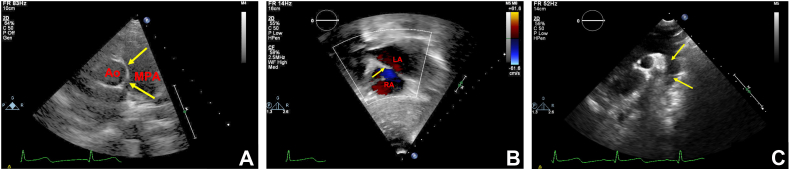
(A) Two-dimensional TTE (5 MHz transducer) high right parasternal view demonstrates intact septation between the aorta and MPA *(arrows)*. (B) Subcostal coronal view with color flow Doppler demonstrates the intact atrial septum *(arrow)*. (C) Suprasternal sagittal view demonstrates a widely patent aortic arch *(arrows)*. *LA*, Left atrium; *MPA*, main pulmonary artery; *RA*, right atrium.
